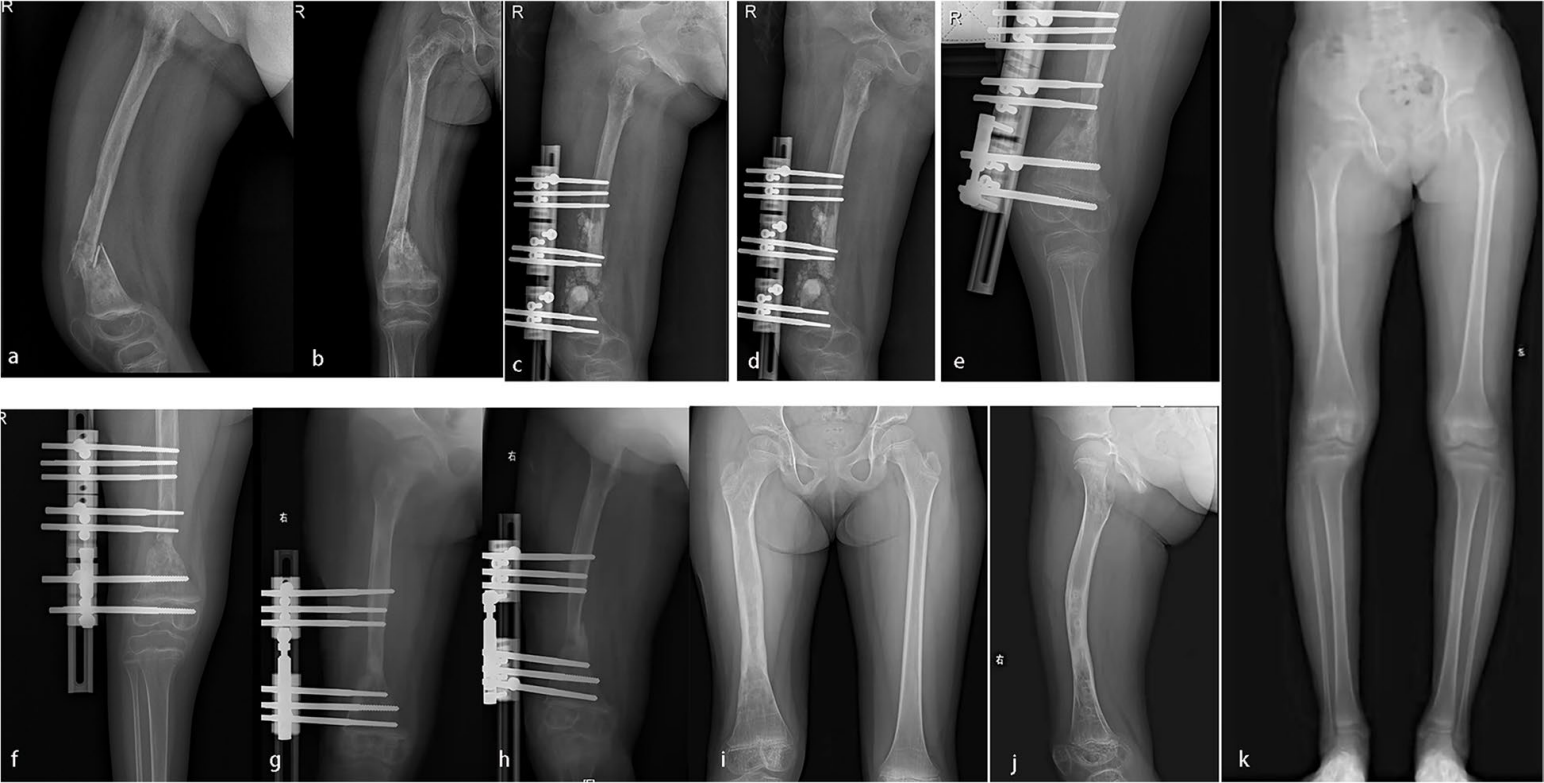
a-b: A five-year-old girl suffered right distal femoral HO and pathological fracture. c-d: Vancomycin impregnated CS was used to fill the void left by decompression and debridement, and the bone transport technique was applied. e–f: Bone transport was finished 3 months postoperatively. g-h: Removing of the distal screw and adjusting the external fixation device. i-k: X-ray examination indicated retardation of bone growth and lower limb discrepancy 19 months postoperatively
